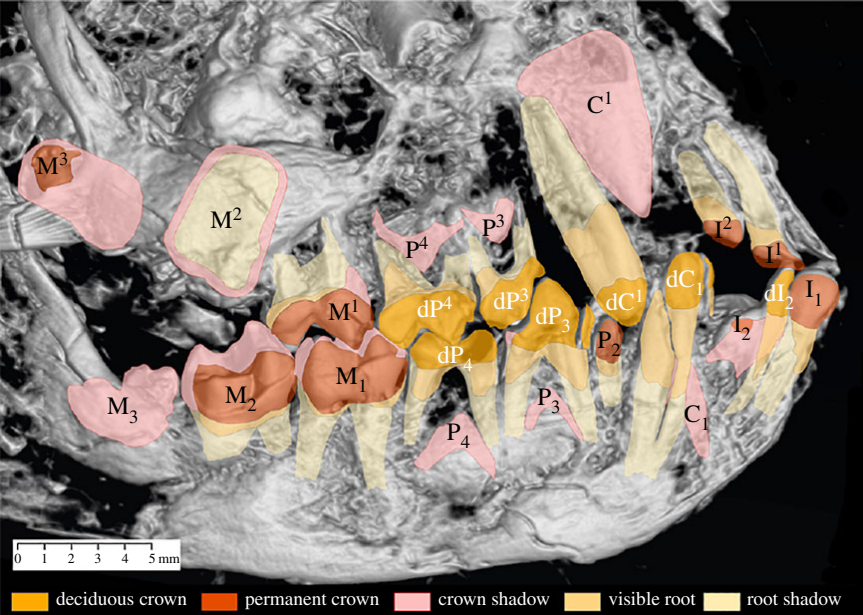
Radiograph of the right side of the skull of *Darwinius masillae* showing the deciduous (indicated with a ‘d’) and permanent teeth. Adapted from Franzen *et al*. [17], fig. 5.
